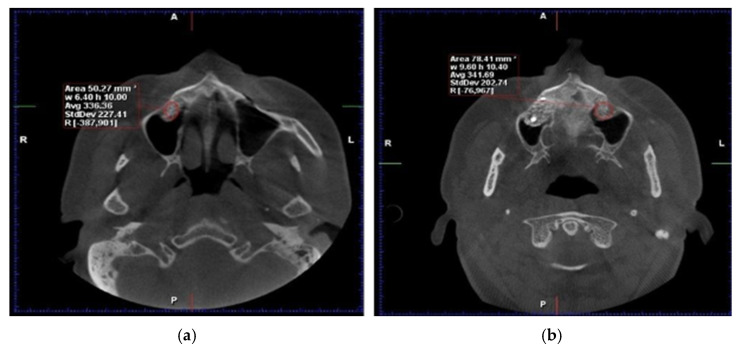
Axial CBCT reconstructions. Evaluation of HU density six months post−intervention: (a) with RS administration, (b) without adjuvant therapy involving RS (Personal Collection).
